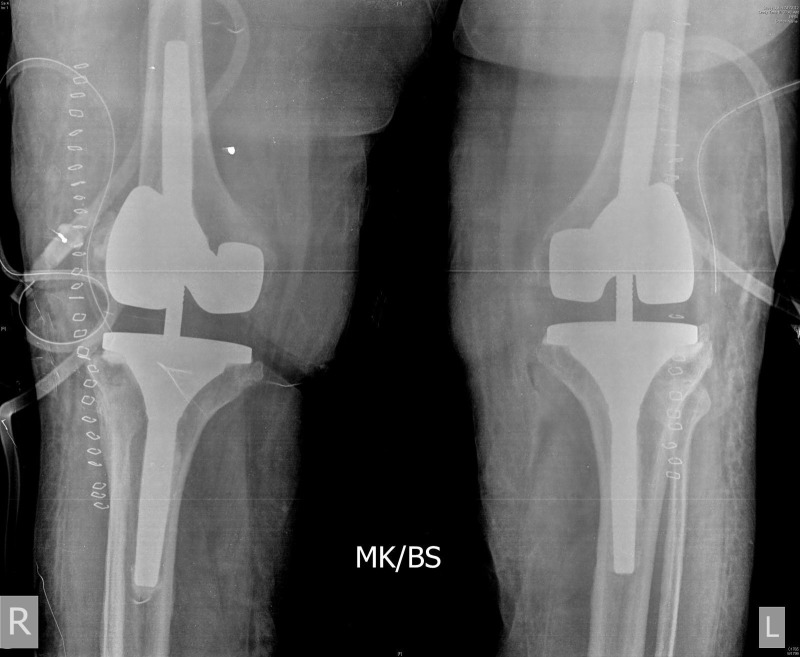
Postoperative AP radiographs after bilateral revision total knee arthroplasties showing well aligned new constrained implants in both knees.
